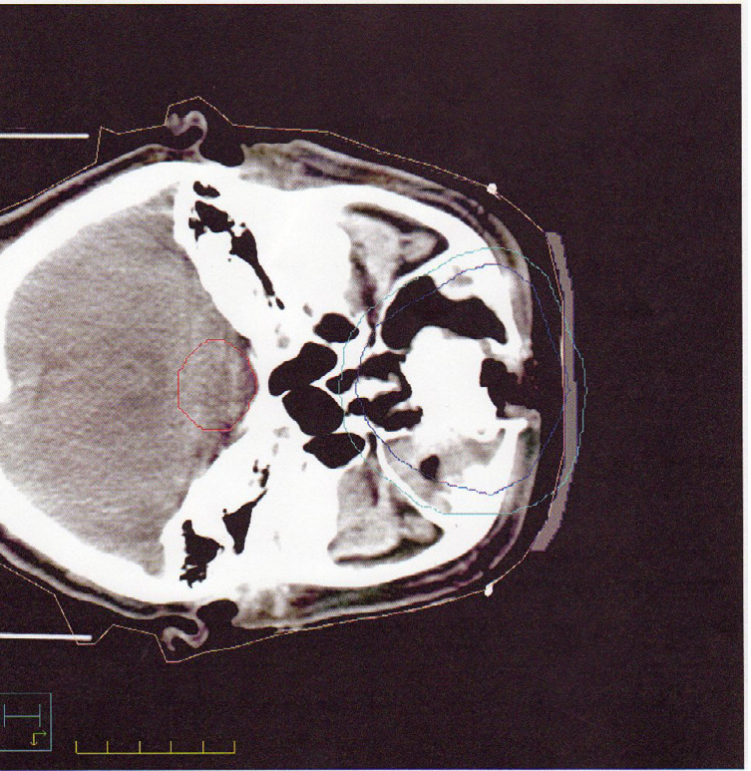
CT Scan showing area of sqaumous cell carcinoma.
